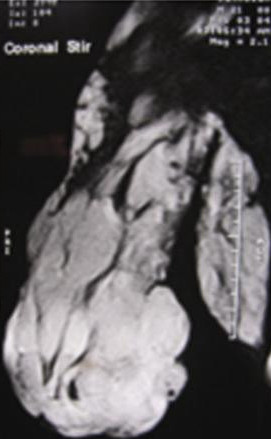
Magnetic resonance imaging scan multiple dilated veins in the superficial aspect of the right upper limb with bone hypoplasia.
